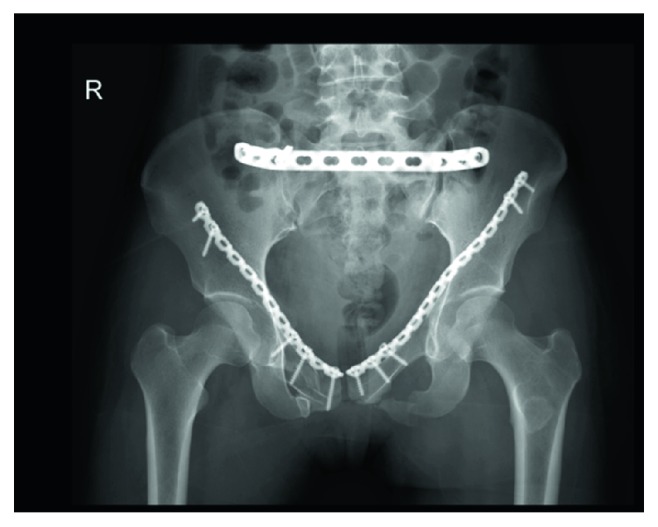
The X-ray image of the pelvis with the laparoscopic-assisted plate.
